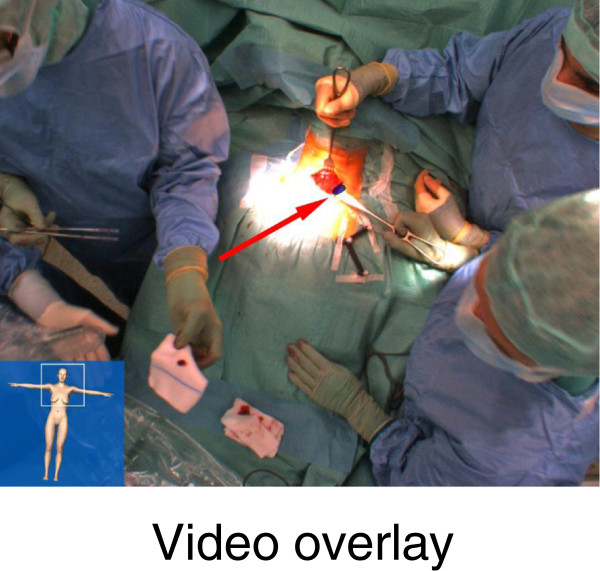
Freehand SPECT of the left side of the neck of patient 2 as video overlay. The arrow shows the adenoma in the image.
